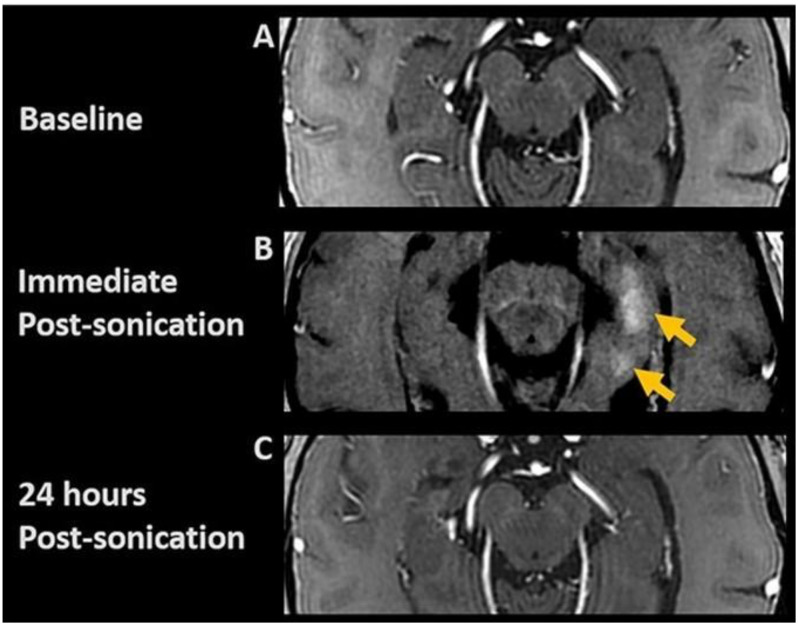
Post-contrast axial T1-weighted images. (A) Baseline; (B) Immediate post-FUS treatment demonstrating contrast enhancement (arrows) at target sites, indicating focal BBB opening; (C) 24-h post-sonication images show resolution of parenchymal contrast enhancement, indicating BBB closure.
